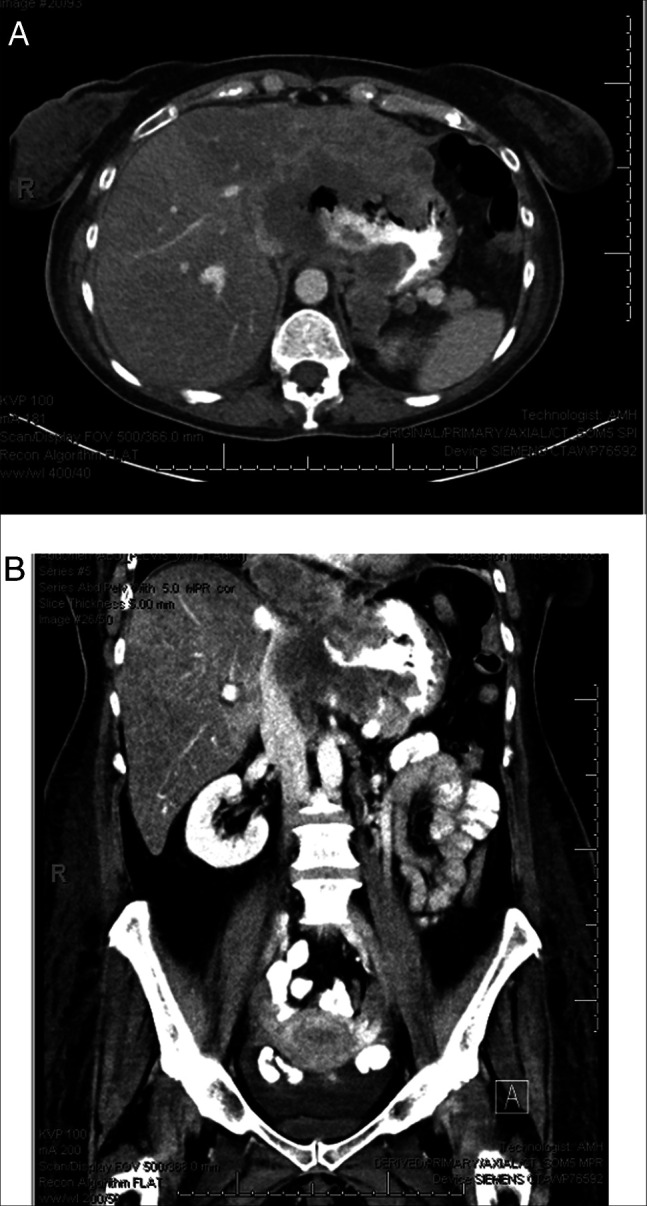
(A) CT image axial view of the enlargement of the GE junction/gastric fundus malignancy with central necrosis in addition to oral contrast filled channels extending rightward from the distal esophagus to the gastric fundus. (B) CT image coronal view of the enlarged GE junction/gastric fundus malignancy with oral contrast extending from the distal esophagus to the gastric fundus. GE, gastroesophageal.
